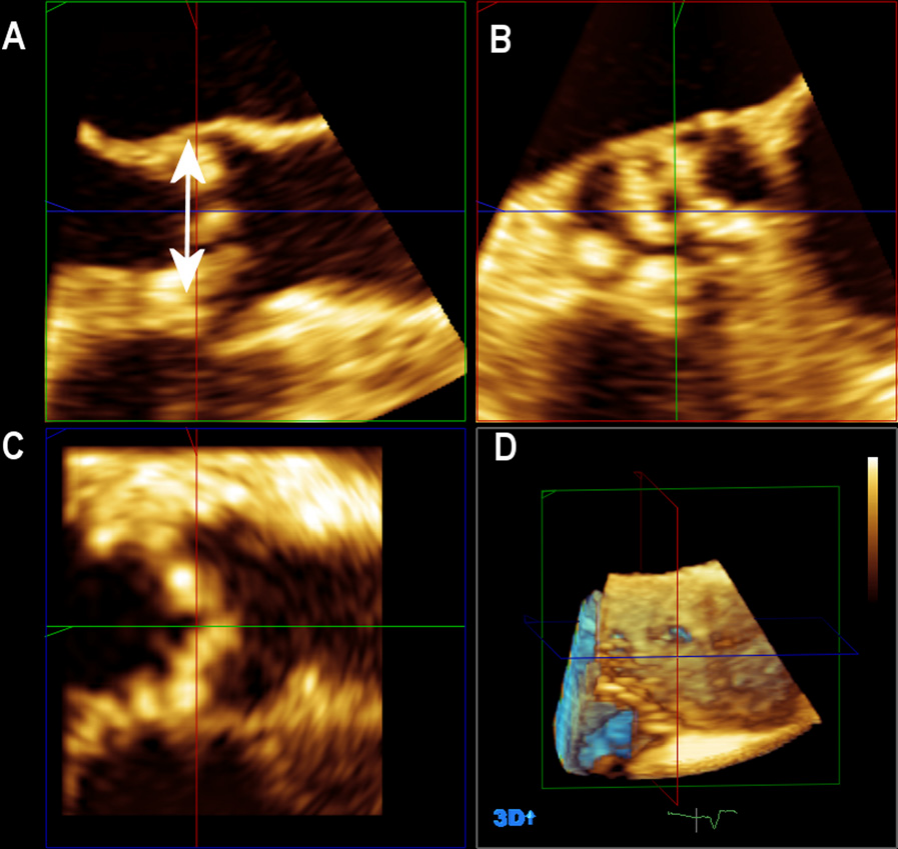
Reconstruction of the three-dimensional transesophageal echocardiography in 3-chamber view (A,D) and measurement of aortic annulus diameter (A). The coronal and the short axis views of the aortic valve are shown (B, C).
